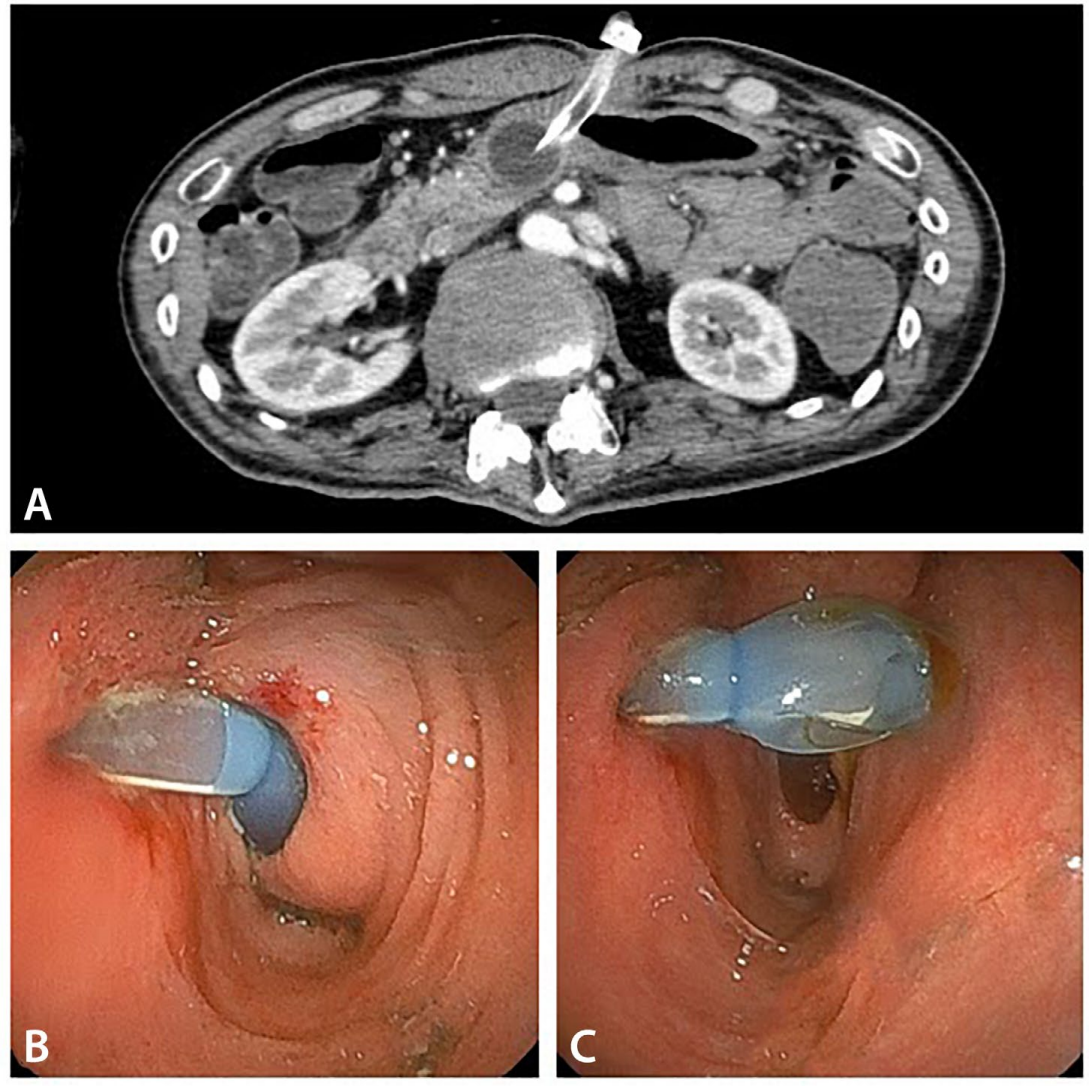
Imaging findings of PEG tube malposition. (A) Abdominal CT showing the PEG tube tip in the first portion of the duodenum. (B) Endoscopic image showing the PEG balloon tip malpositioned beyond the pylorus. (C) Endoscopic image showing the PEG balloon tip repositioned in the stomach.
